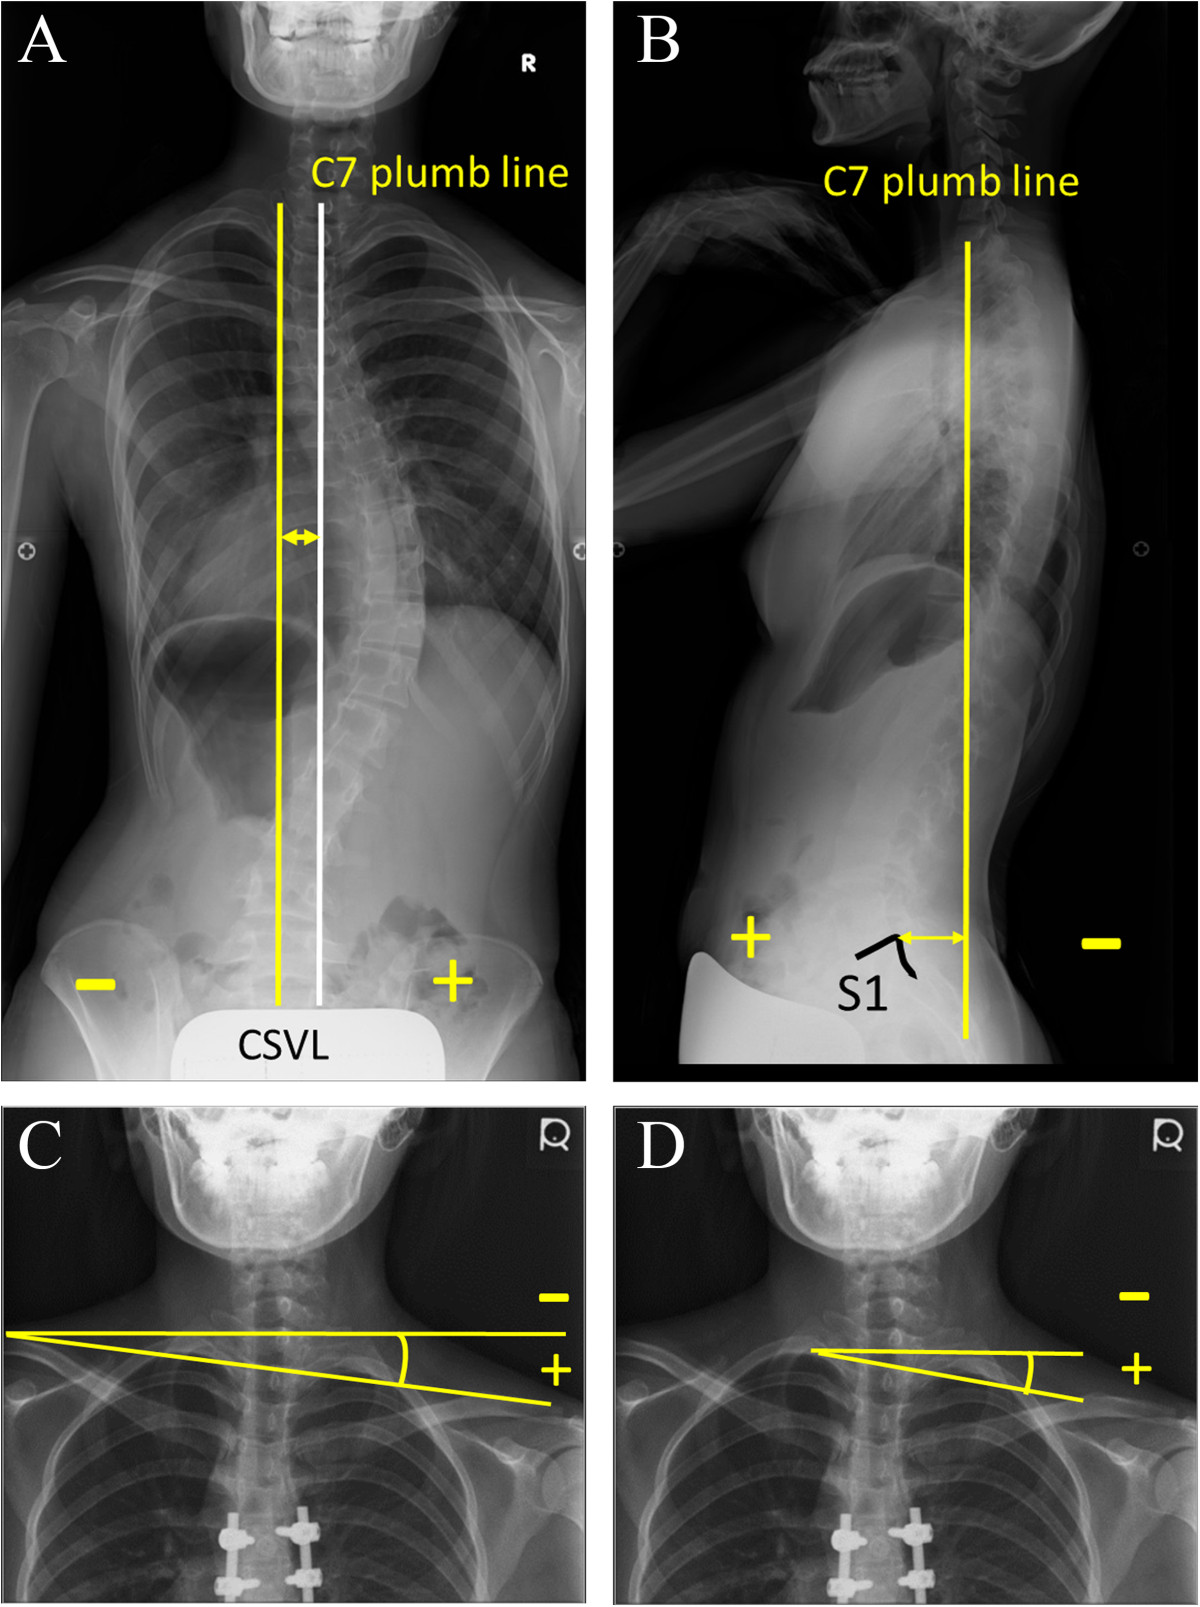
Radiographic measurements. A. Coronal balance; a distance between the central sacral vertical line and the C7 plumb line (right; positive, left; negative) in the standing postero-anterior radiograph. B. Sagittal balance: a distance between the C7 plumb line and the posterosuperior corner of the sacrum in the lateral standing radiograph. (anterior; positive, posterior; negative). C. Clavicle angle: the angulation of a horizontal line and the tangential line connecting the highest two points of each clavicle (left side up; positive, left side down; negative). D. T1 tilt angle; the angulation of the upper endplate of T1 to the horizontal line (left side up; positive, left side down; negative).
